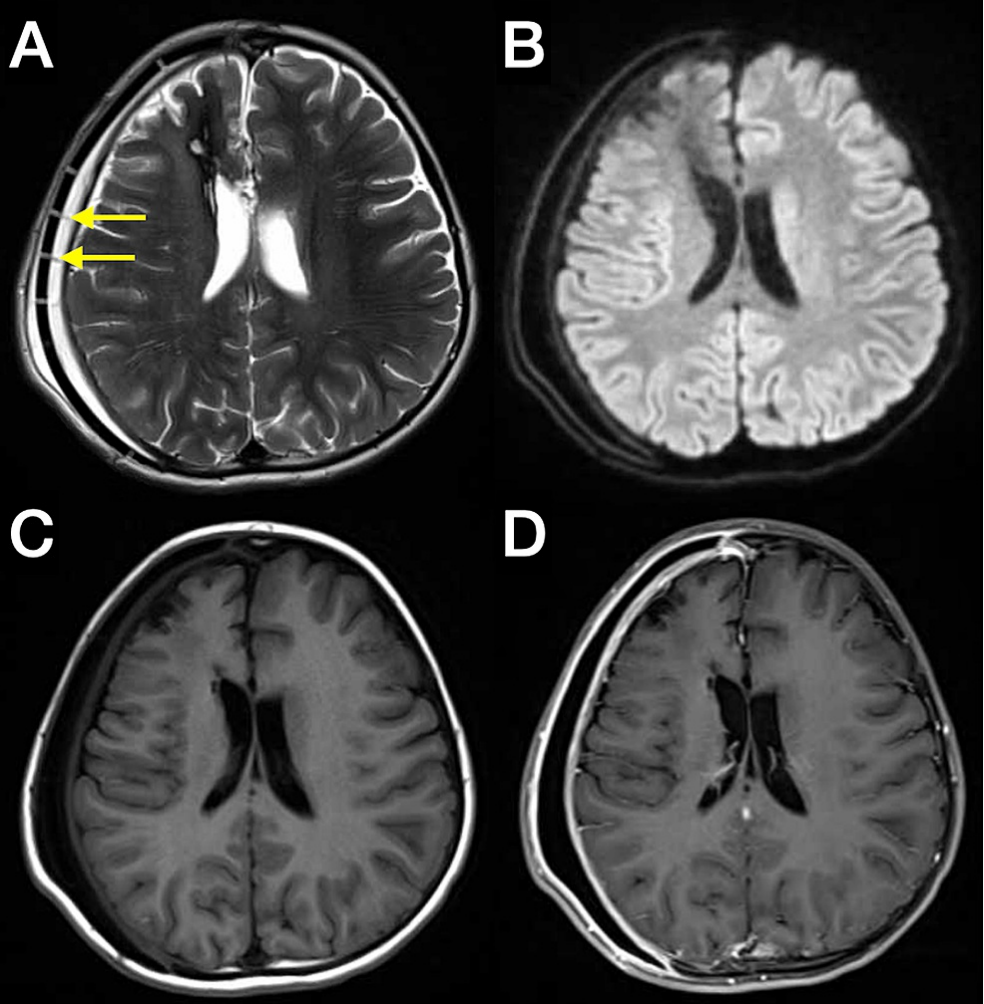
Brain MRI with and without gadolinium contrast three weeks after the PEEK cranioplasty Brain MRI with and without gadolinium contrast three weeks after the polyetheretherketone (PEEK) cranioplasty demonstrated both epidural as well as subgaleal fluid collections. (A) Isointense columnar structures extending from the inner surface of the galea to the epidural surface (arrows) within hyperintense fluid signal; (B) diffusion weighted sequence with hypointense subgaleal and epidural fluid signal, inconsistent with infection; (C) pre- and (D) post-contrast T1 with smooth, homogeneous enhancement of the dura and galea, felt to be more consistent with reactive than infectious etiology.
